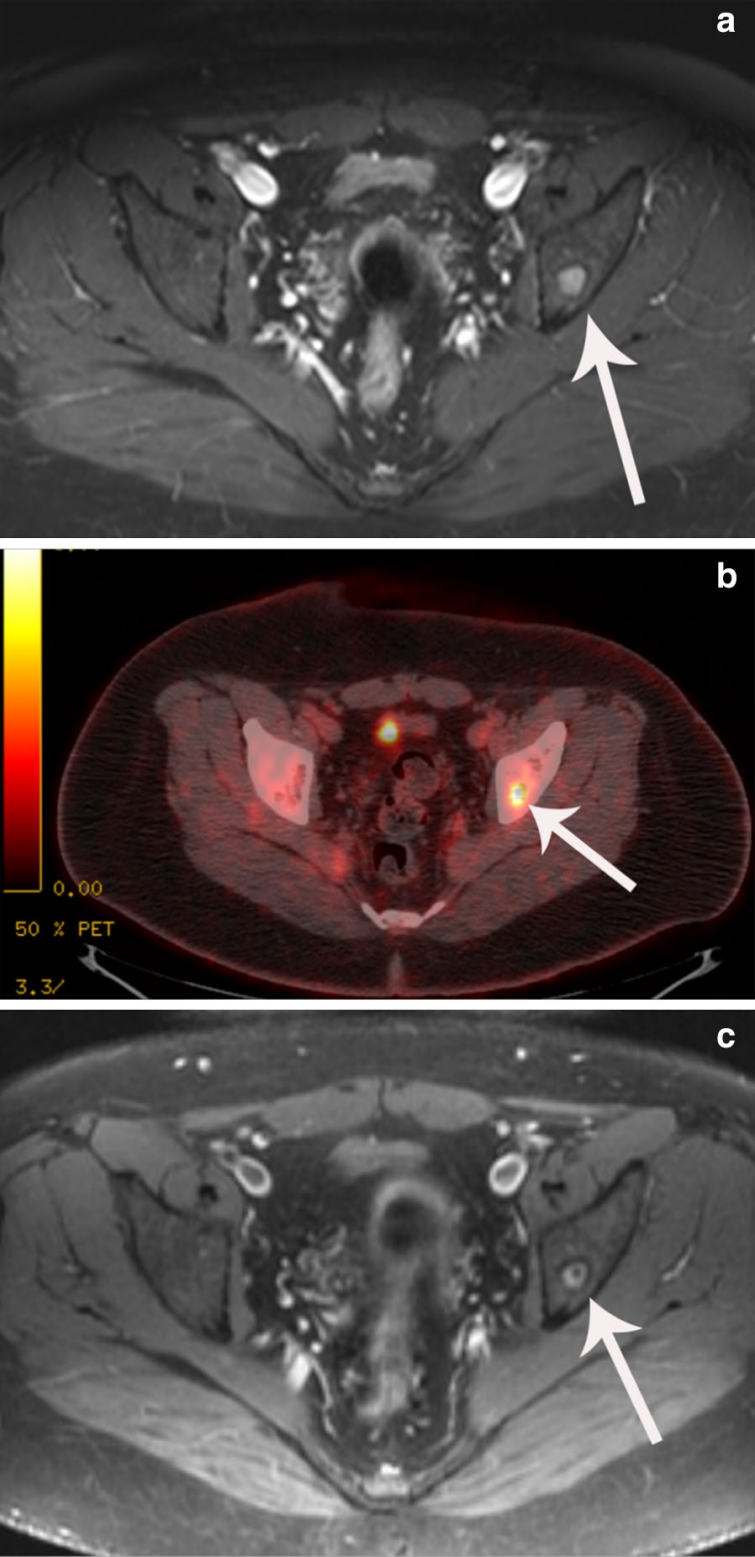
Imaging studies obtained during treatment for a 22-year-old man (“Case 2”) with pseudomyogenic hemangioendothelioma. a Fat-saturated T1-weighted axial magnetic resonance image with intravenous contrast enhancement of the pelvis demonstrates a solitary enhancing tumor focus in the supra-acetabular region of the left iliac bone (*white arrow*), before everolimus treatment. b Positron emission tomography-computed tomography fusion image through the pelvis demonstrates corresponding metabolic activity. This image was obtained before the patient began everolimus treatment. c Fat-saturated T1-weighted axial MR image with intravenous contrast enhancement of the pelvis obtained after 2 months of everolimus demonstrates partial response of the solitary enhancing tumor focus in the supra-acetabular region of the left iliac bone
